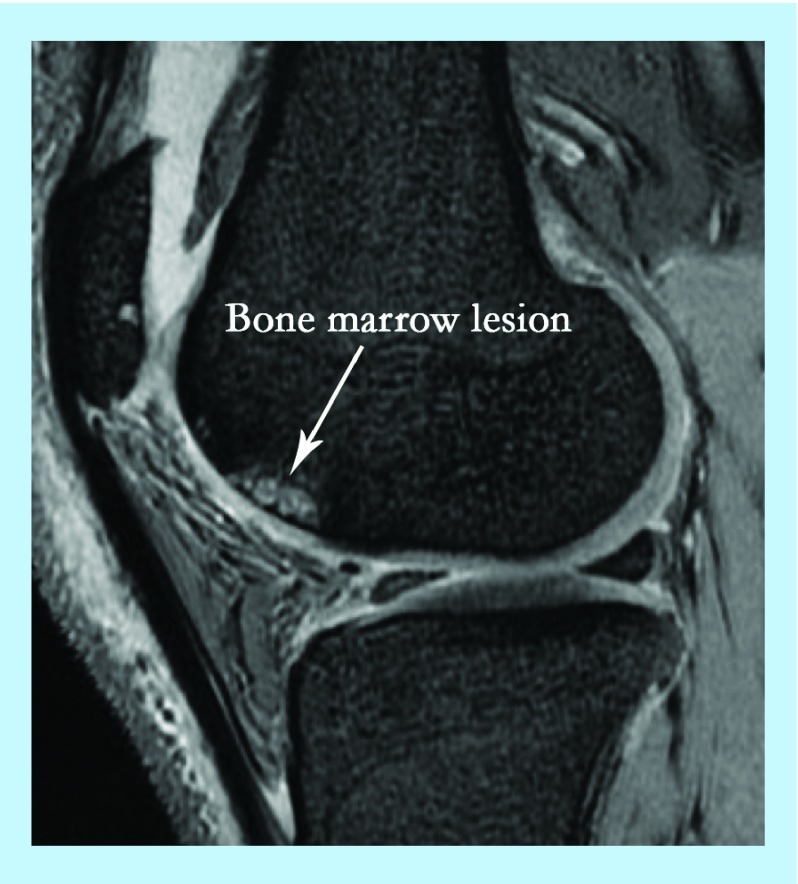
MRI of knee osteoarthritis showing bone marrow lesion.
